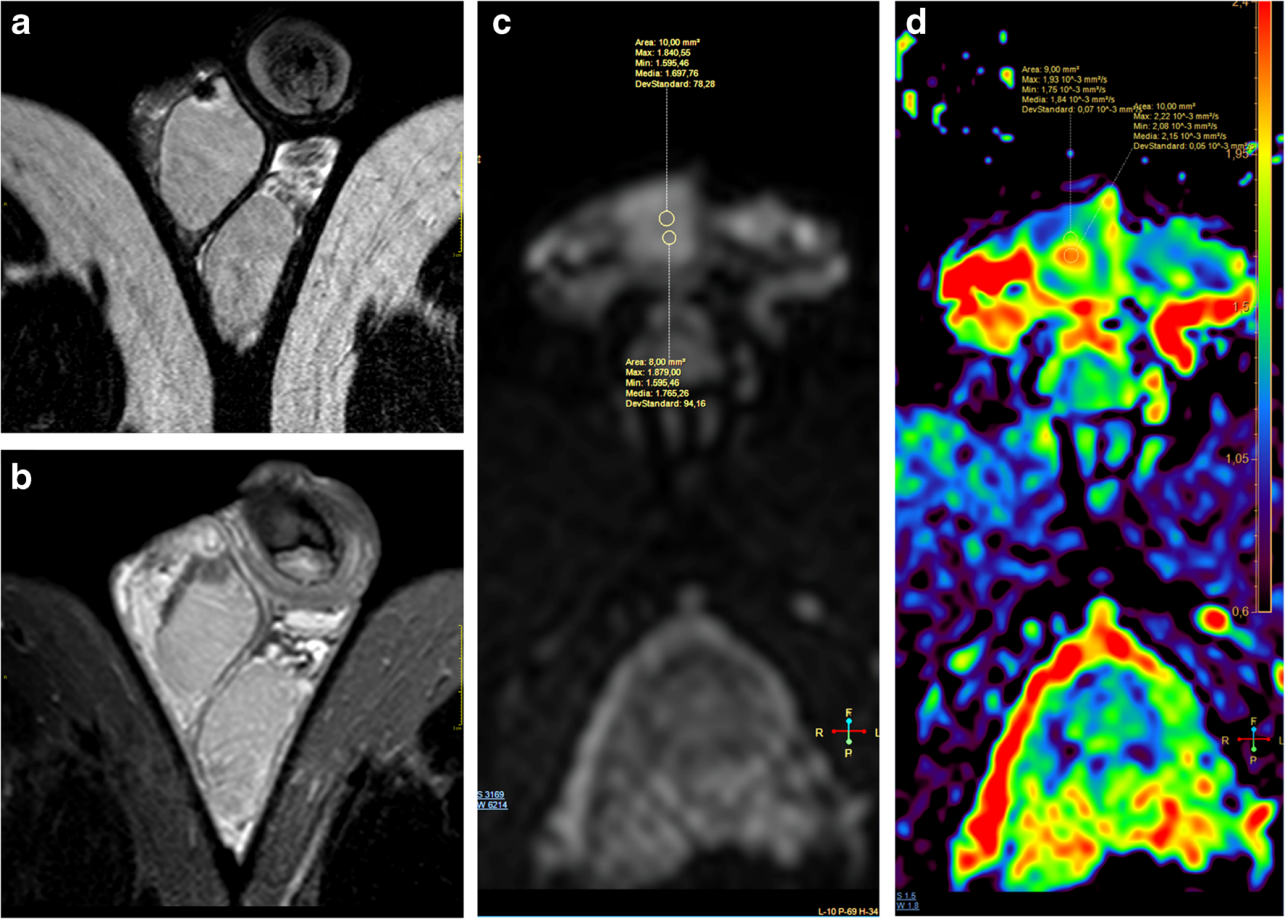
a-d Hypoechogenic lesion at the site of previous tumour enucleation (Leydig cells tumour). MRI allowed the identification of an area of fibrosis. a T2-weighted TSE sequence, coronal view; b postcontrast T1-weighted SPIR TSE sequence; c-d. ADC map
